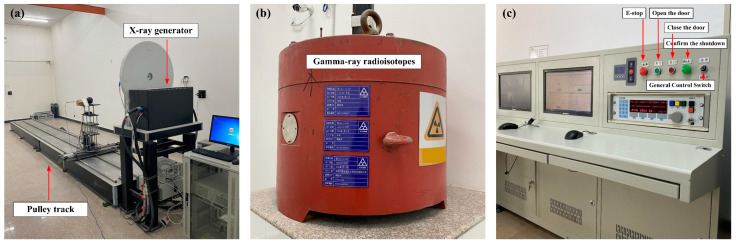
(a) Standard radiation field X-ray reference standard radiation device. (b) γ radiation source device. (c) Console.
